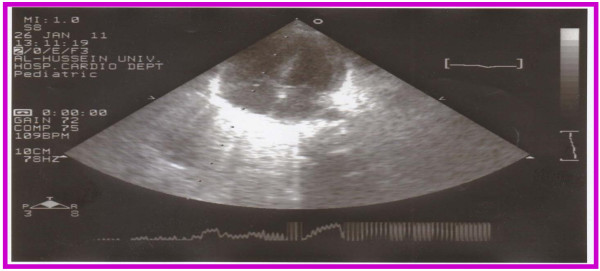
Apical four chamber view two-dimensional echocardiogram showing septal hypertrophy in an infant of diabetic mother.
